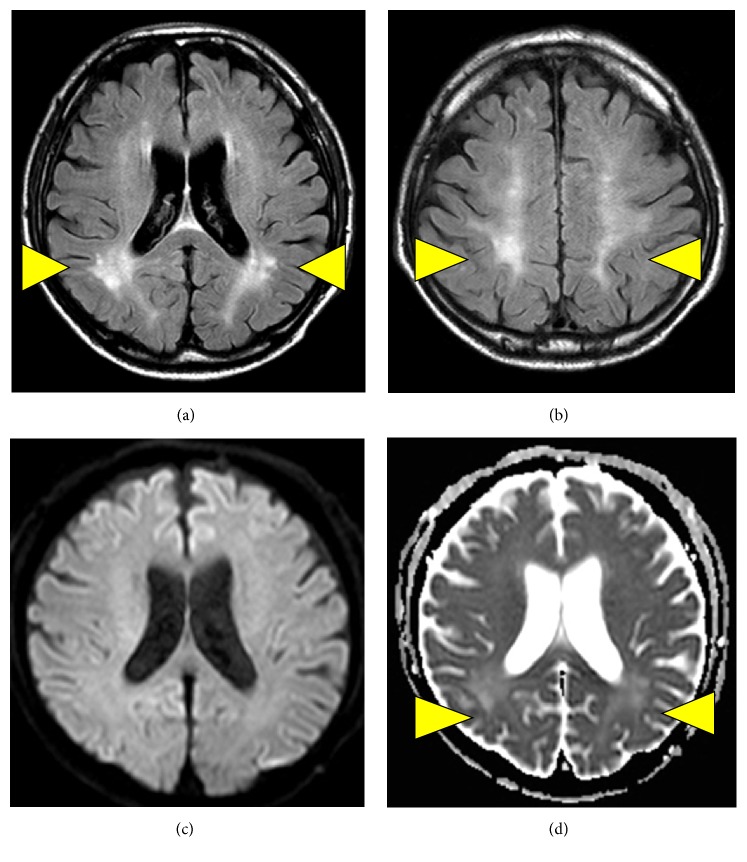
Cranial MRI taken on day 15, showing brain edema. ((a), (b)) T2-weighted FLAIR MRI showing increased signal intensities bilaterally in the cortical and subcortical areas of the occipital lobe (arrowhead). (c) DWI demonstrates no hyperintense signal alterations in these regions. (d) ADC is elevated in bilateral occipital lesions (arrowhead).
